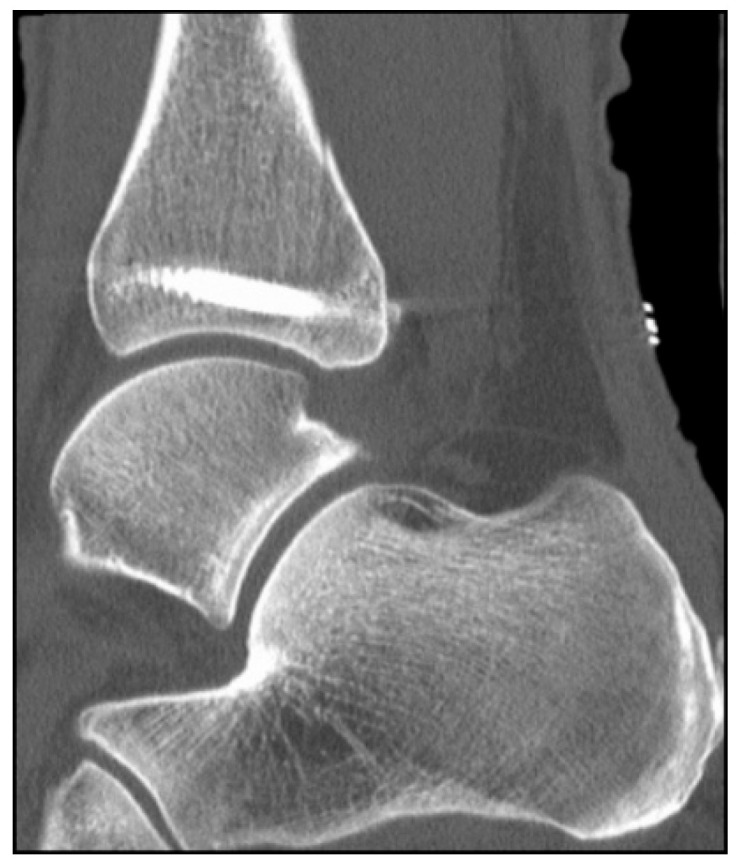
Postoperative CT scan demonstrating optimal screw position and anatomical reduction.
